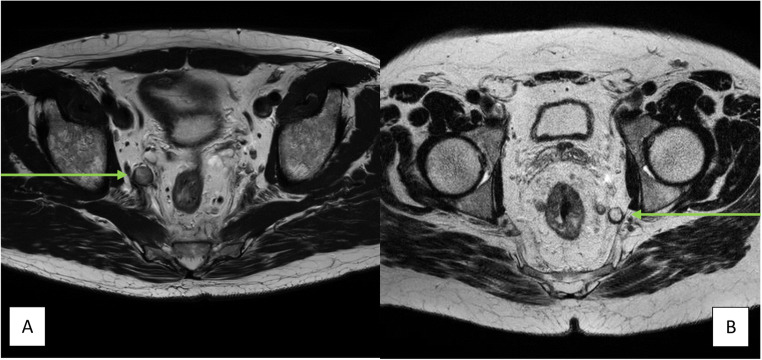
A, B Two examples of an enlarged and suspicious lateral lymph node on axial T2-MRI. Left (A): enlarged lateral lymph node (green arrow) located medial of the main trunk of the internal iliac artery and is therefore located in the internal iliac compartment. Right (B): enlarged lateral lymph node (green arrow) located caudal of where the main trunk of the internal iliac artery exits the pelvis and is therefore located in the obturator compartment
